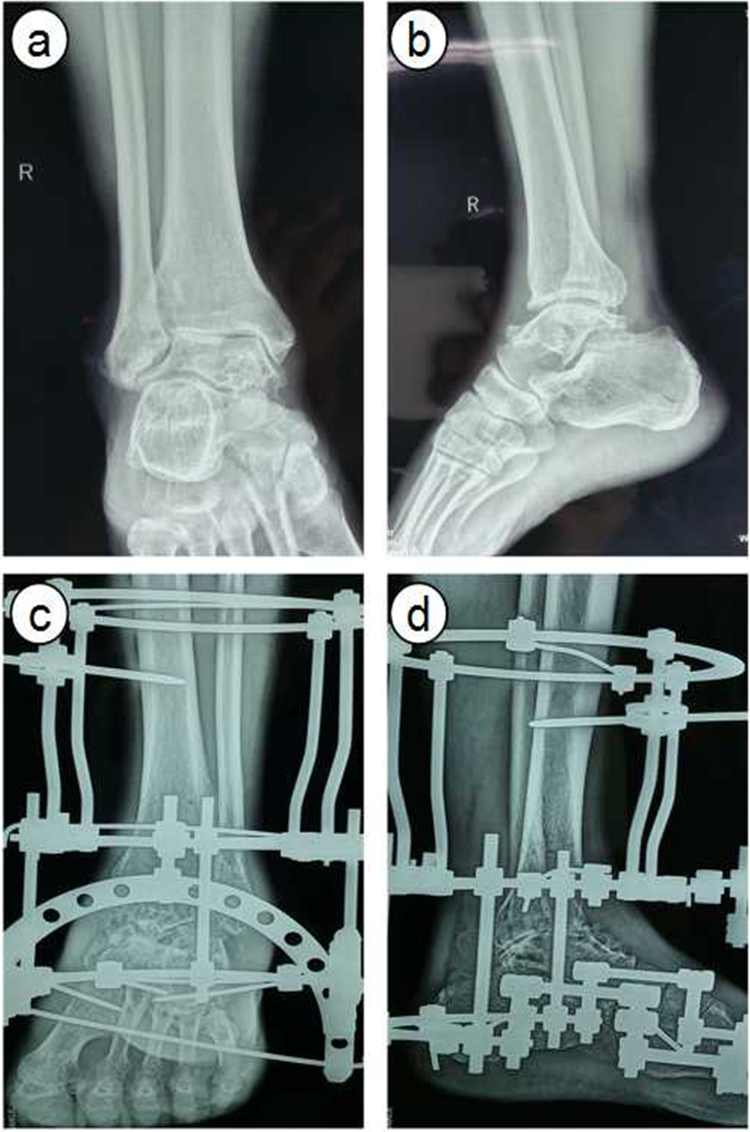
Radiographs showing a 57 yrs patient with end-stage ankle arthritis underwent Ilizarov external fixation. (a) and (b) pre-operative anteroposterior and lateral radiographs, (c) and (d), post-operative 3 month anteroposterior and lateral radiographs.
